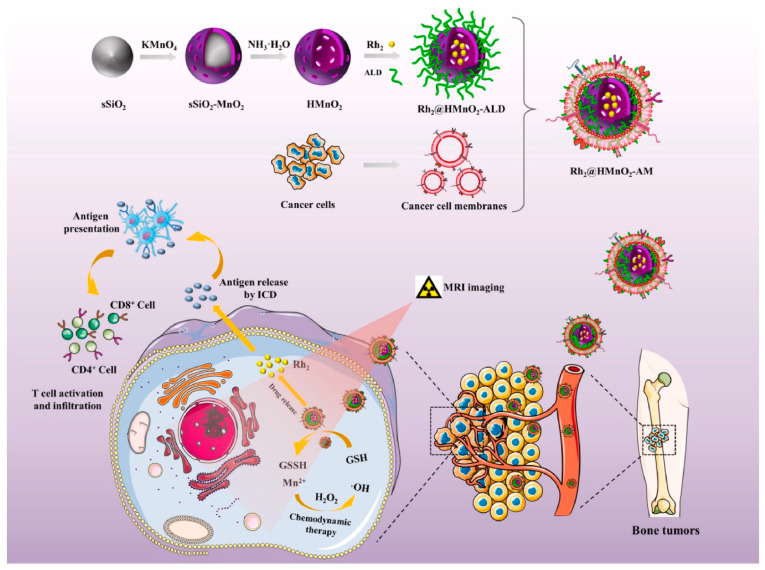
Rh2@HMnO_2_-AM synthesis procedure and the mechanism of MRI-guided immuno-chemodynamic synergistic osteosarcoma therapy. Reprinted from an open-access source [162].
